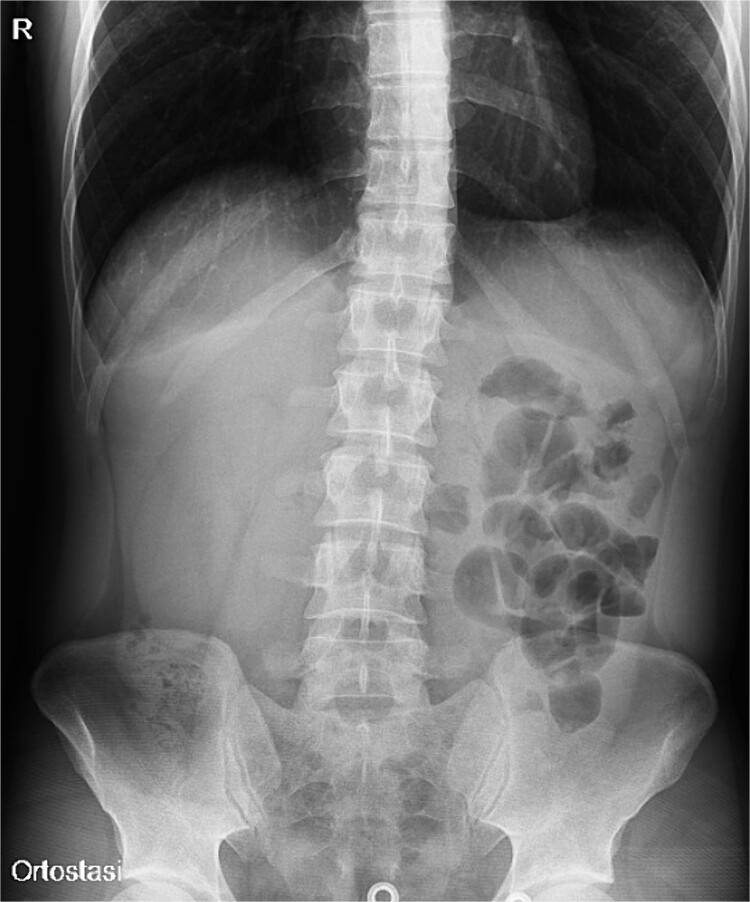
Plain abdomen X-ray showing limited visibility of the colon on the right side with small bowel loops’ distension on the left regions, without free air.
